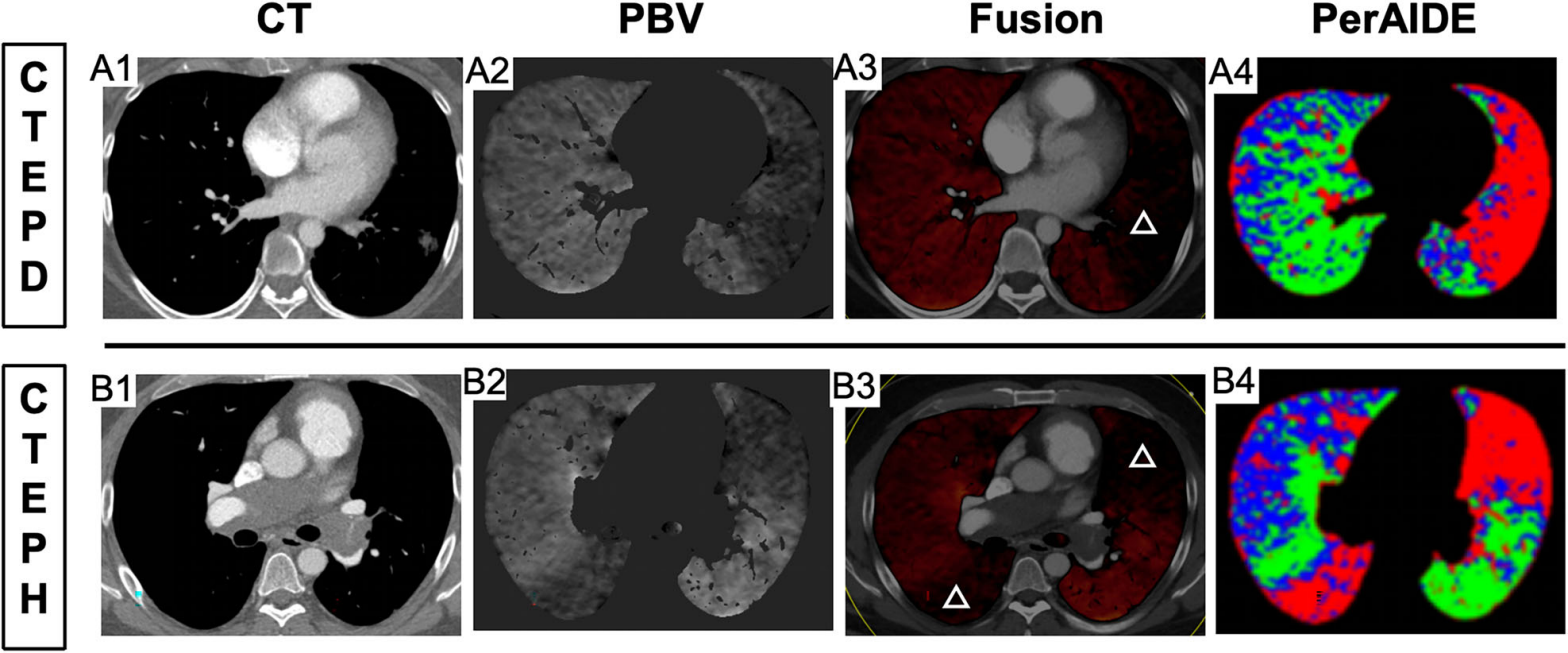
Representative images of CTEPD and CTEPH. A1–A4 refer to images from the same slice of a typical CTEPD patient. B1–B4 refer to images from the same slice of a typical CTEPH patient. (A1) The CT image of CTEPD; (A2) The lung PBV image of CTEPD; (A3) The CT/PBV fusion image of CTEPD; (A4) The final image generated by the PerAIDE system of CTEPD. (B1) The CT image of CTEPH; (B2) The lung PBV image of CTEPH; (B3) The CT/PBV fusion image of CTEPH; (B4) The final image generated by the PerAIDE system of CTEPH. White triangles in A3 and B3 represent the perfusion defects area. In A4 and B4, the perfusion defects are areas in the pulmonary vasculature where there is a total absence of blood flow (red regions). Areas with decreased perfusion have reduced blood flow intensity (blue regions), but not a complete absence. Normally perfused areas have entirely normal blood flow (green regions). CTEPD, chronic thromboembolic pulmonary disease; CTEPH, chronic thromboembolic pulmonary hypertension; PBV, perfusion blood volume; PerAIDE, pulmonary perfusion artificial intelligence automatic quantification analysis system based on DE-CTPA
